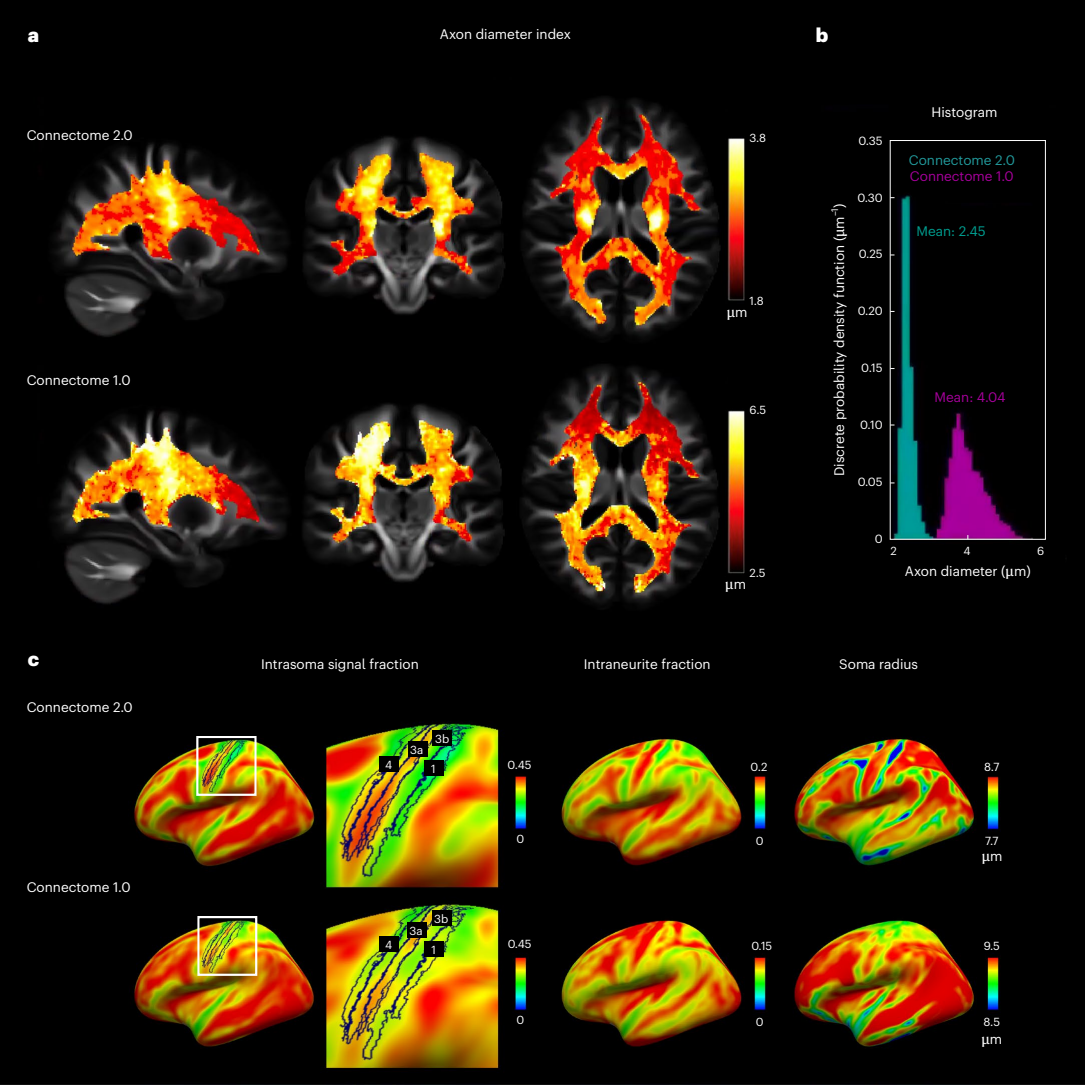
In vivo human brain tissue microstructure results. White and grey matter microstructural estimates obtained from biophysical modelling of diffusion MRI data acquired in 10 age- and sex-matched healthy adults scanned on Connectome 2.0 and Connectome 1.0 scanners. a, Axon diameter index maps estimated from the AxCaliber-SMT model averaged across individuals. b, Histogram of axon diameter indices from Connectome 2.0 and Connectome 1.0 extracted from a representative region of interest in the posterior corona radiata. c, Estimates of intrasoma signal fraction (left), intraneurite signal fraction (centre) and soma radius (right) from fitting of the SANDI model. SANDI estimates were averaged across individuals and projected onto the cortical surface. Inset: intrasoma signal fraction demonstrates estimates within sensorimotor cortex, delineated as Brodmann areas 4, 3a, 3b and 1.
